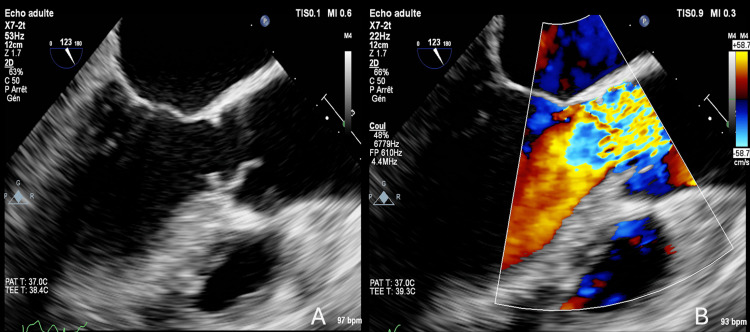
(A) Midesophageal long-axis view showing a discrete subaortic membrane in the LVOT, located 5 mm below the aortic valve. (B) Midesophageal long-axis view with color Doppler demonstrating obstruction in the LVOT. LVOT: left ventricular outflow tract.
